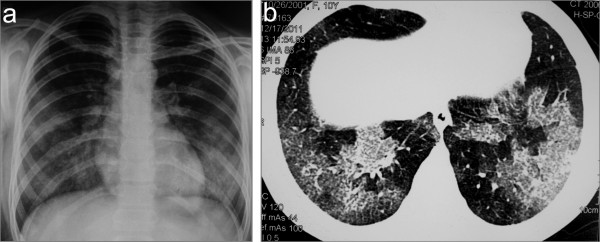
Chest X-ray (a) and CT scan (b) showing bilateral lung infiltrates as a result of diffuse alveolar hemorrhage.
